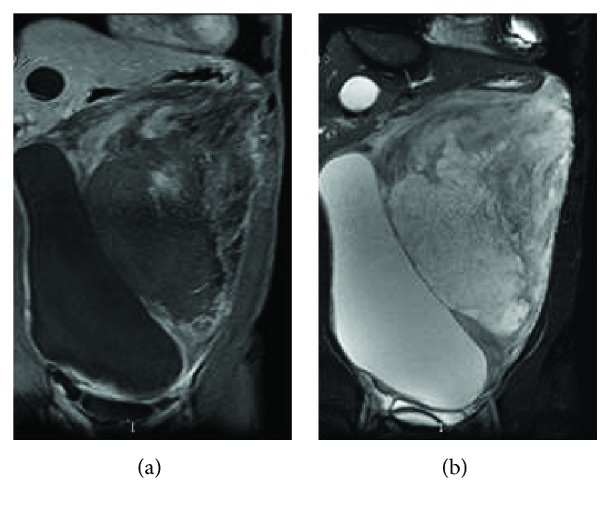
(a) MRI (coronal view, T1W1): tumor had generally low signal density, but some parts had high signal density. (b) MRI (coronal view, T2W1): tumor had high signal intensity.
